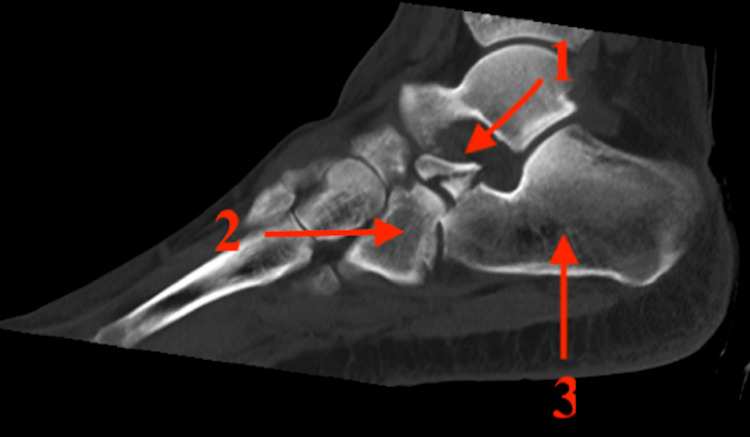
CT sagittal view of the left ankle 1: Anterior process of the calcaneus; 2: cuboid; and 3: calcaneum.
